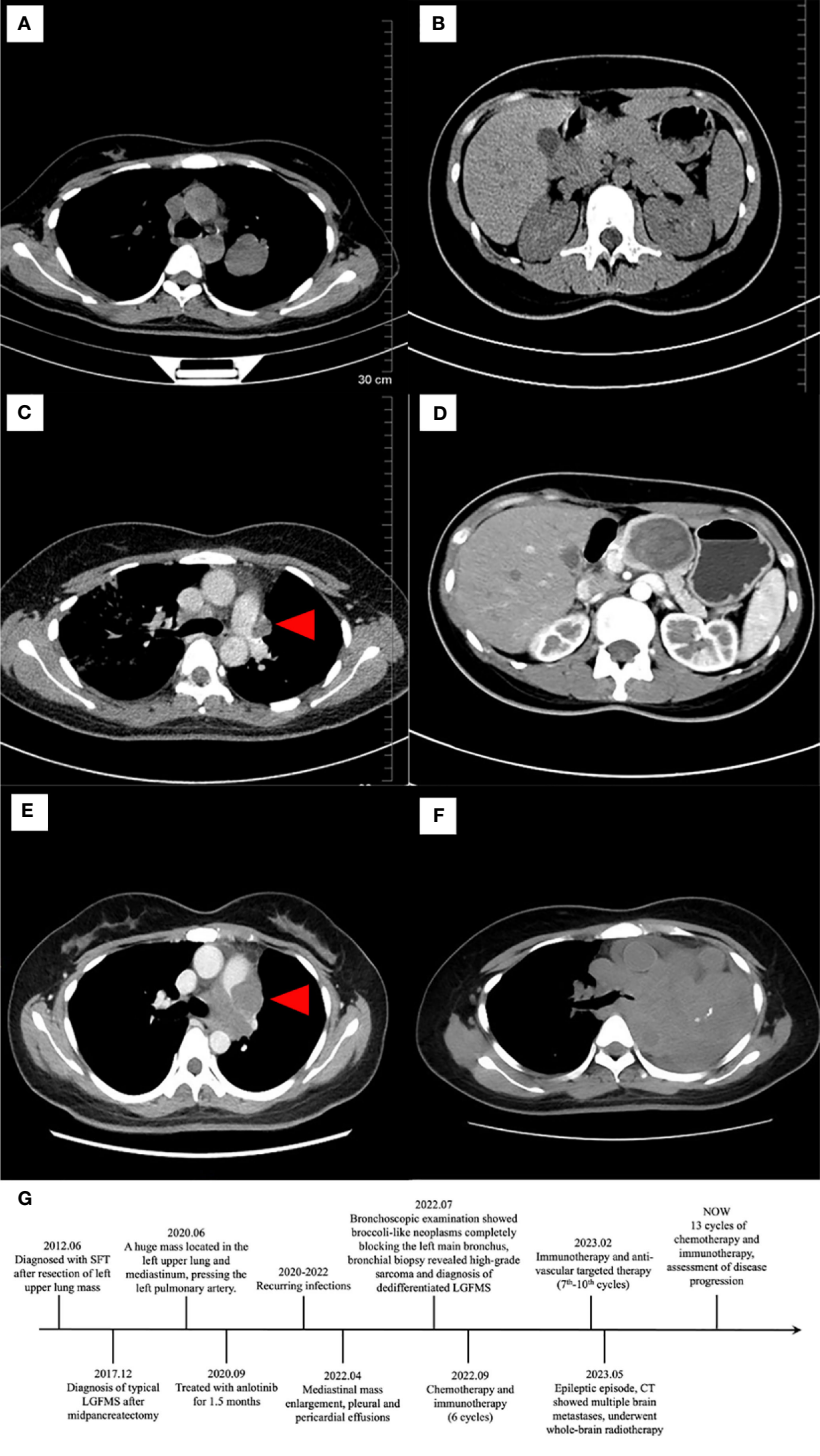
Computed tomography (CT) of lung (left) and pancreas (right). In 2012, CT showed a mass in left upper lobe (A). No lesion was seen in the pancreas (B). In 2016, CT found a small rise at the left edge of the main pulmonary artery ((C), red arrowhead). Then in 2017, CT revealed a low-density mass measuring 60mm×50mm×30 mm in the body of pancreas, the mass being mildly enhanced and homogeneous, the pancreatic duct dilatated, and the splenic artery slightly compressed (D). In 2020, there was a tumor in the left middle and upper mediastinal mass measuring 70mm×56mm×53mm, pressing the left pulmonary artery ((E), red arrowhead). In 2022, on the left side of the mediastinum, a mass-like soft tissue mass was seen, with a larger size than the lesion in 2020 (F). The timeline of the patient’s clinical history is presented (G).
